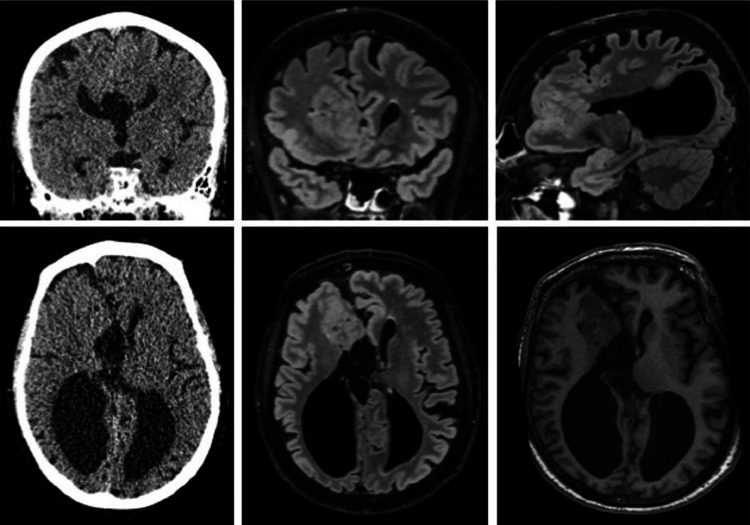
Brain CT from 2019, soft-tissue coronal and axial images (top right and bottom right); Brain MRI from 2023, T2-FLAIR coronal, sagittal and axial images (middle and top left), and T1 axial image (bottom left), showing continuous layer of gray matter in antero-medial aspect of the right frontal lobe extending from the ventricular wall to cortex and causing bulge on the ventricular wall without intervening CSF cleft or white matter suggesting transmantle heterotopia. Colpocephaly and agenesis of the corpus callosum agenesis are also present.
